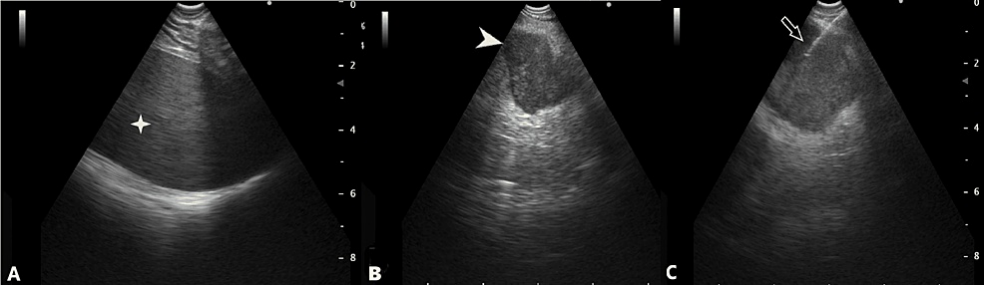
Endobronchial ultrasound images with the transducer placed in the stomach showing the spleen A: The sliding counter-clockwise and caudally left adrenal gland is visible (indicated by the star), B: Bulky adrenal is visible (marked by the arrowhead), C: Needle (marked by the arrow) placed inside the adrenal gland
